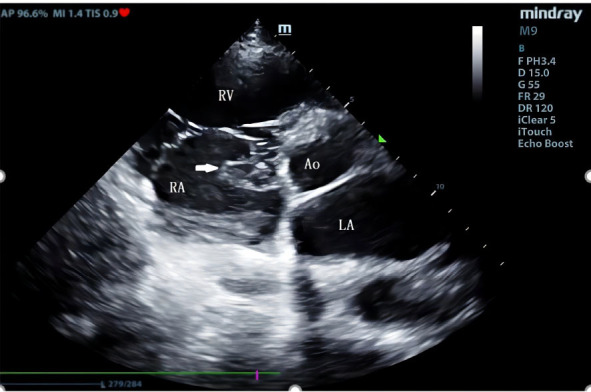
Male, 55 years old with suspected high-risk pulmonary embolism. Transthoracic echocardiography showed direct signs of thrombus in the right heart system, at the short axis level of the aorta. A thrombus was visible in the right atrium. (RV: right ventricle, RA; right atrium, LA: left atrium, Ao: aorta; arrow indicates thrombus).
